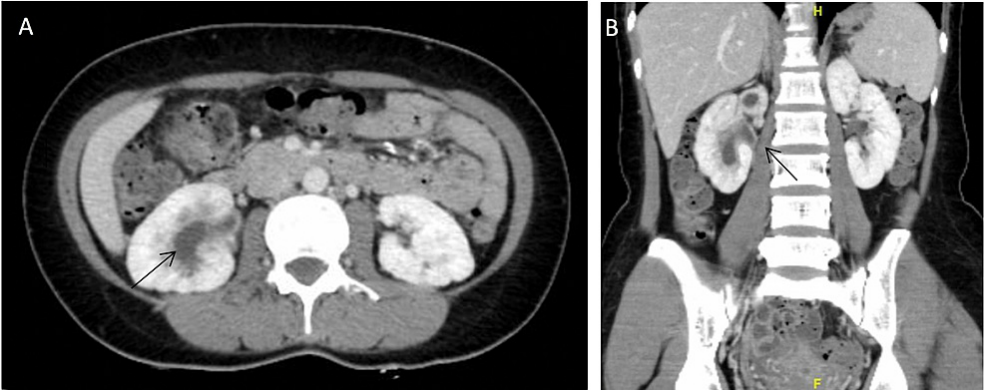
Axial (A) and coronal (B) contrast-enhanced computed tomography images of the abdomen and pelvis subsequently revealed right kidney multiple dilated calyces and renal pelvis, mild proximal dilatation of the right ureter, thickening of the renal pelvis and right ureteric wall (arrows), and mild diffuse thickening of the urinary bladder wall.
